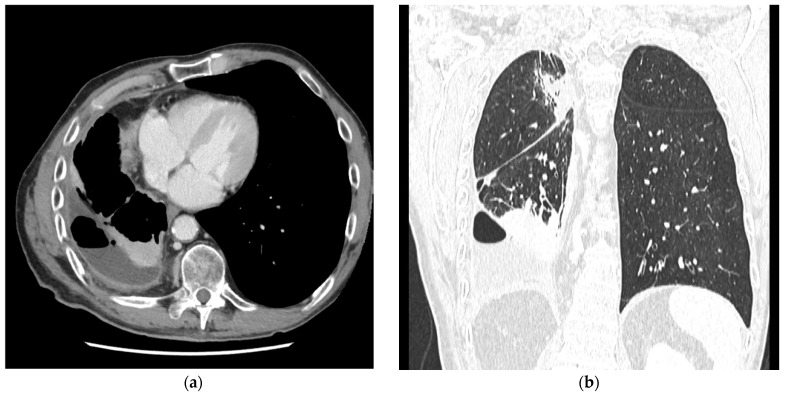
(a,b) Computed tomography sections demonstrating posterolateral hydropneumothorax due to non-expandable lung in a patient with pulmonary adenocarcinoma and pleural involvement.
